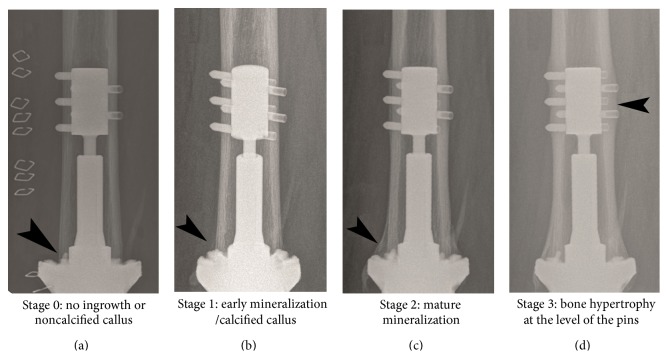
Radiographic stages of osseointegration according to the new assessment system.
